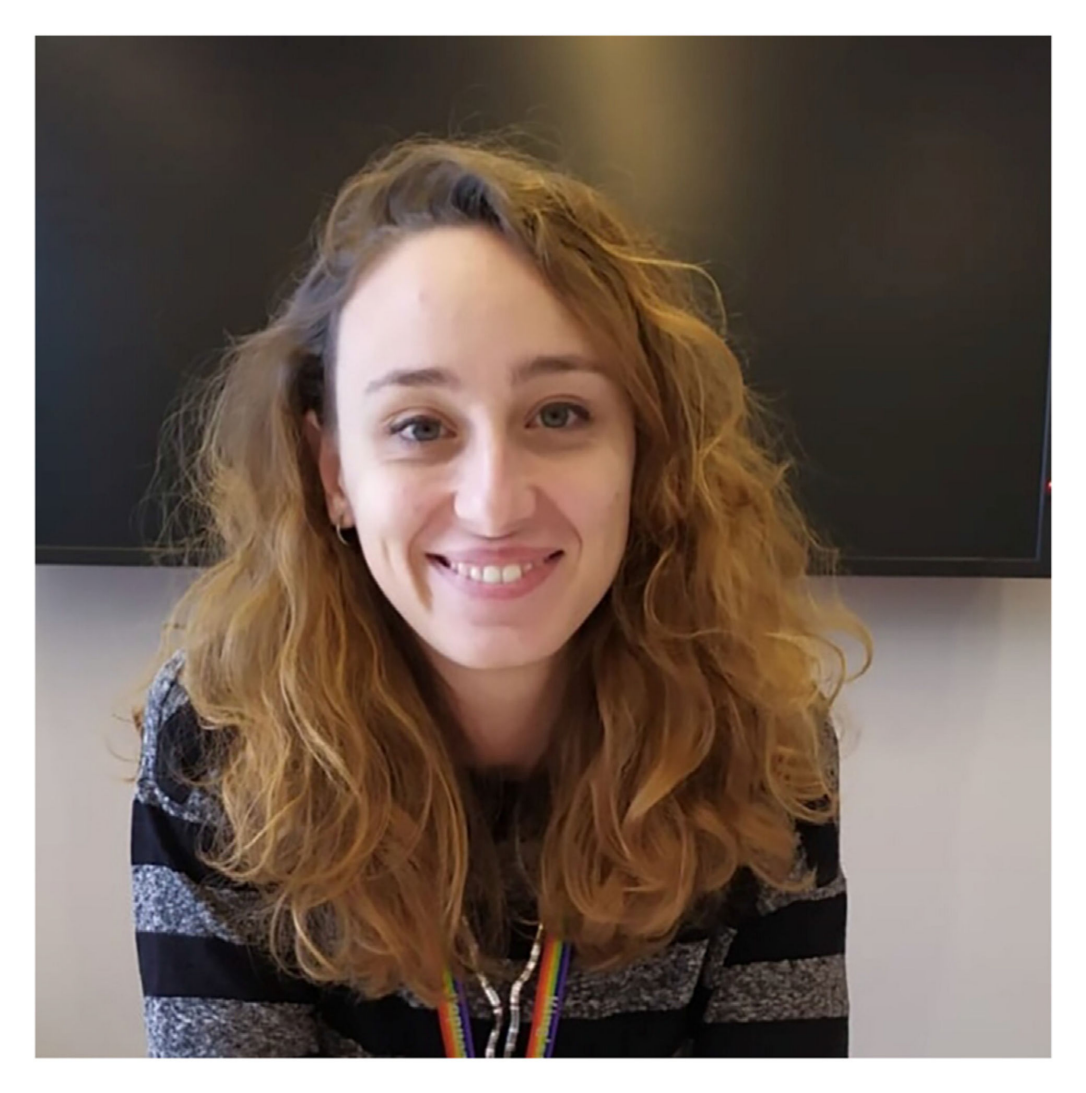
Maria Antonietta Nettis Dr Maria Antonietta Nettis is an academic psychiatrist with a strong interest in the interplay between body and mind in psychiatric conditions. As a psychiatrist, she currently works at the National Psychosis Unit, Bethlem Royal Hospital. As a researcher, she is part of the Research & Development Department committee at South London and Maudsley, NHS Trust, where she contributes to clinical research studies delivery. Dr Nettis has recently completed her PhD in Psychological Medicine at the Institute of Psychiatry, Psychology and Neuroscience, King’s College London. Amongst her research achievements, she was able to demonstrate how a worse immune-metabolic status can predict a poor clinical outcome in first episode psychosis. She also designed a human model of the mechanism of communication between the peripheral and the central immune system, by using PET and MRI to image the neuroinflammatory response to interferon-alpha. Finally, she published data on a RCT with add-on minocycline in patients with treatmentresistant depression and increased levels of inflammation. Currently, Dr Nettis is the local Clinical Principal Investigator for King’s College London in a multi-centre RCT investigating the efficacy of a novel antiinflammatory drug in treatment-resistant depression (Antidepressant Treatment with P2X7Antagonist). For her research work, she received several awards including the First place Mental Health Foundation Research Prize, from the Royal Society of Medicine (2019) and the Robert Kerwin Bursary from the British Association of Psychopharmacology (2020). Dr Nettis is actively involved in the Institute of Psychiatry network promoting diversity and inclusion and gender equality in STEM.
